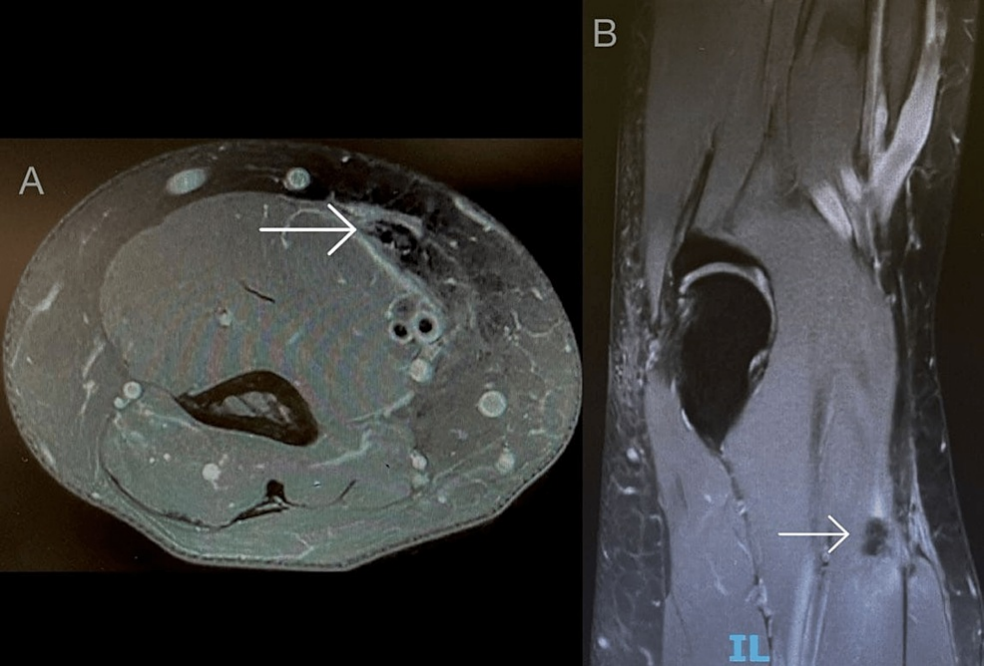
Magnetic resonance imaging findings. (A) Axial T2-weighted image showing the absence of the distal biceps tendon (arrow). (B) Sagittal T2-weighted image showing the retraction of the torn distal biceps tendon (arrow).
